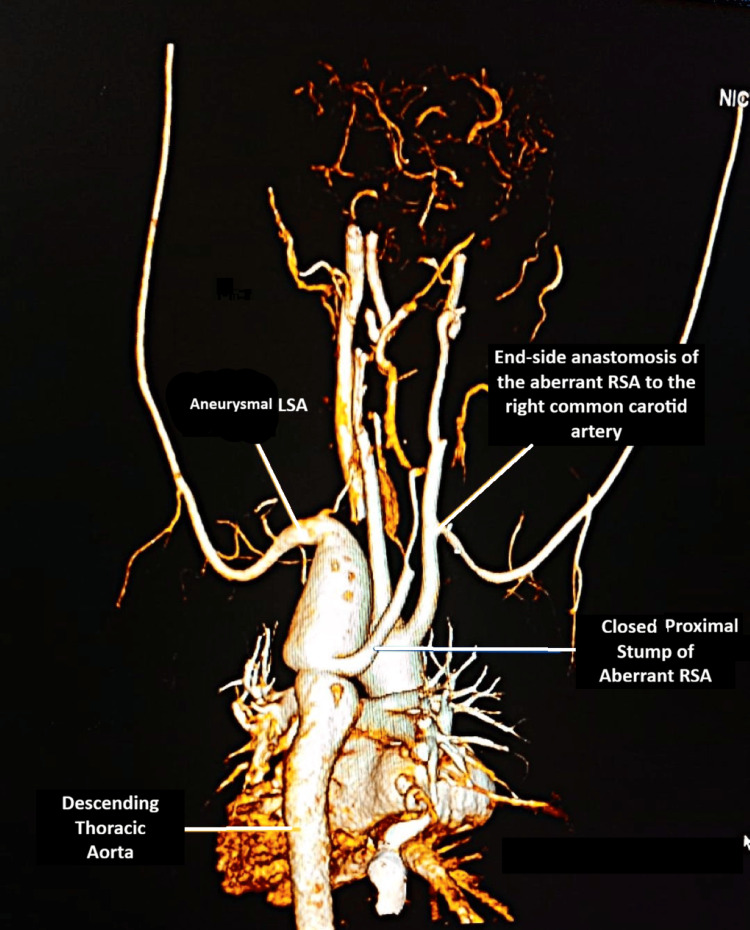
Postoperative three-dimensional computed tomography angiography reconstruction demonstrating successful end-to-side translocation of the aberrant right subclavian artery (RSA) to the right common carotid artery with ligation of the proximal aberrant stump. LSA: left subclavian artery
